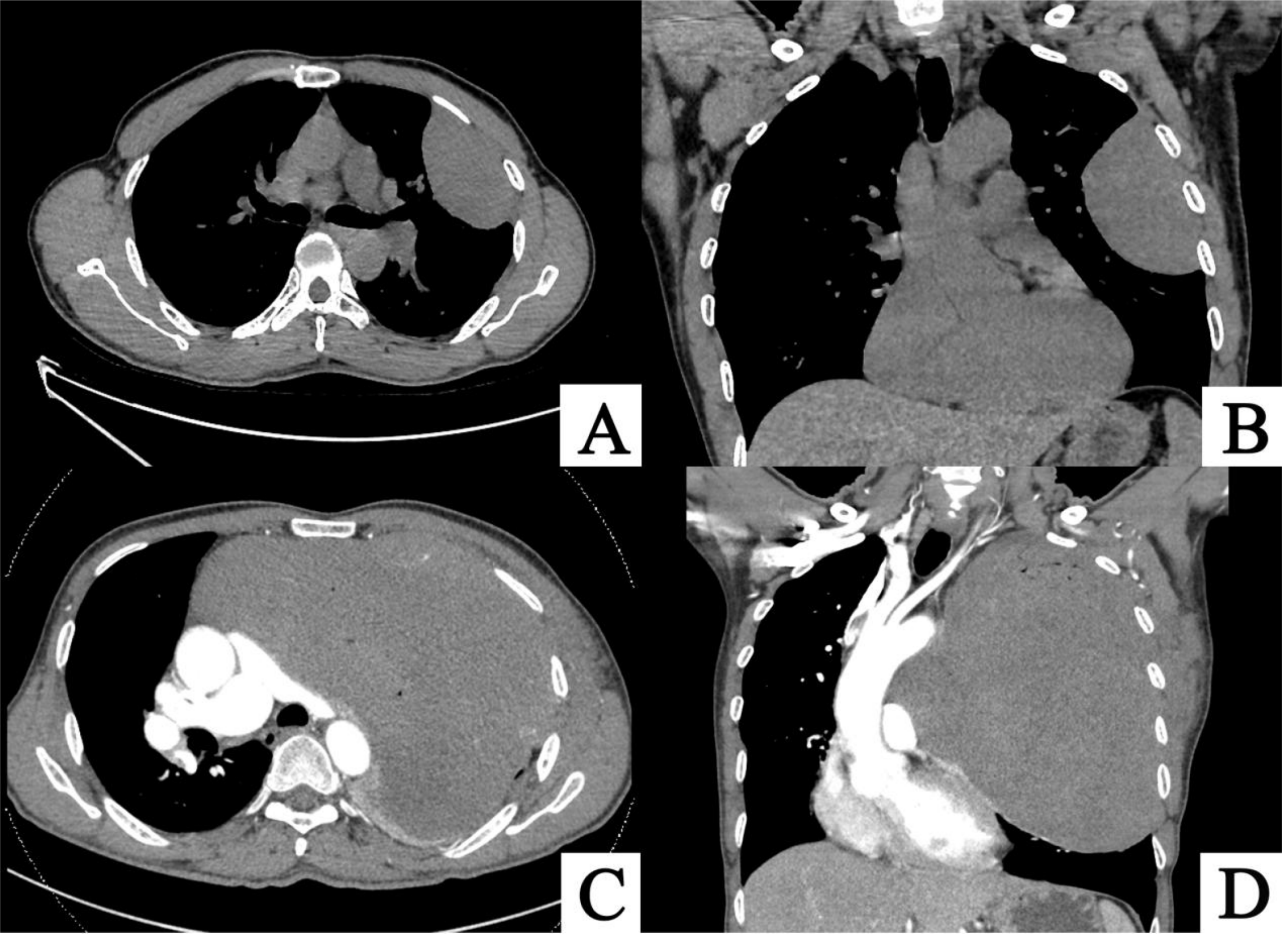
(A, B) CT images from August 19, 2019, showing a spindle-shaped soft tissue density mass in the left thoracic cavity, measuring approximately 7.6 cm × 4.6 cm × 9.1 cm, with compression of the surrounding lung tissue. (C, D) CT images from May 29, 2024, demonstrating a significant increase in the size of the spindle-shaped soft tissue mass in the left thoracic cavity, now measuring approximately 19.2 cm × 13.3 cm × 21.6 cm. The lesion extends toward the mediastinum, causing a rightward shift of mediastinal structures. The main pulmonary artery and right pulmonary artery are compressed and flattened, with notable progression of left lung atelectasis compared to the prior scan.
